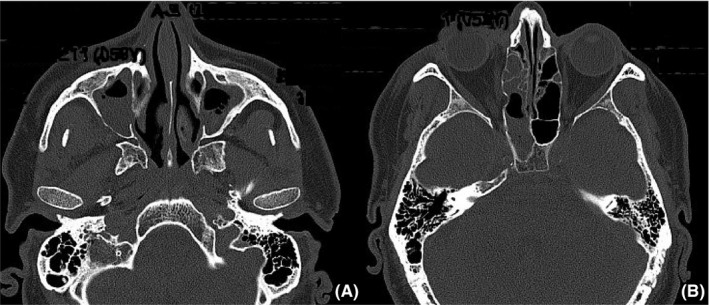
Assial CT study documented opacification of the maxillary sinuses (A), of the right sphenoid sinus and the ethmoidal cells (B)
